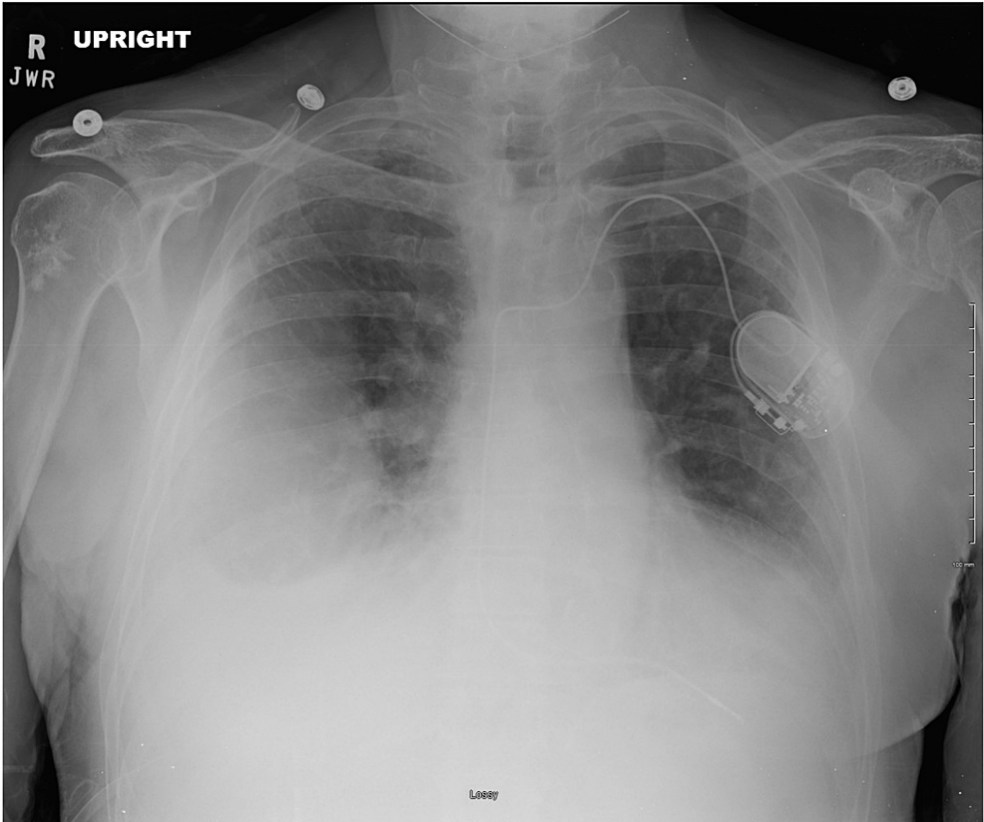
Chest X-ray (2nd Admission)
